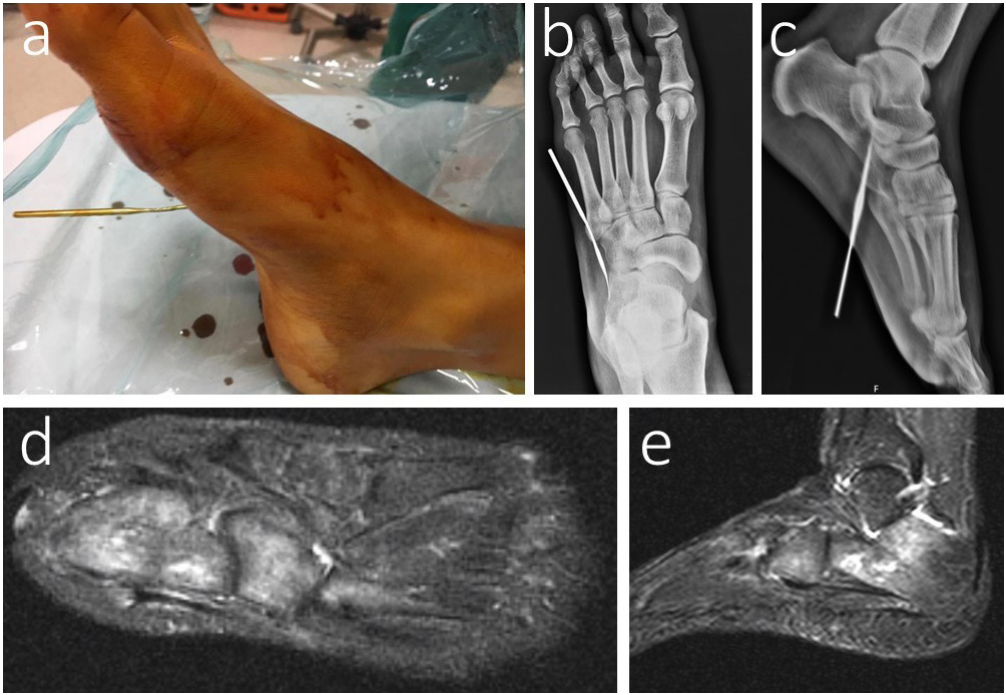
52-year-old female patient with crochet hook injury in her right foot (a); radiographies showed the direction of the crochet hook in her right foot (anteroposterior view (a), lateral view (b)); after removal of the FB, the patient was discharged with oral antibiotics. She had DM as a comorbidity. At 2 weeks follow-up, the MRI-T2 axial view showed osteomyelitis of calcaneus, cuboid, and 5th metatarsal bones (d,e).
